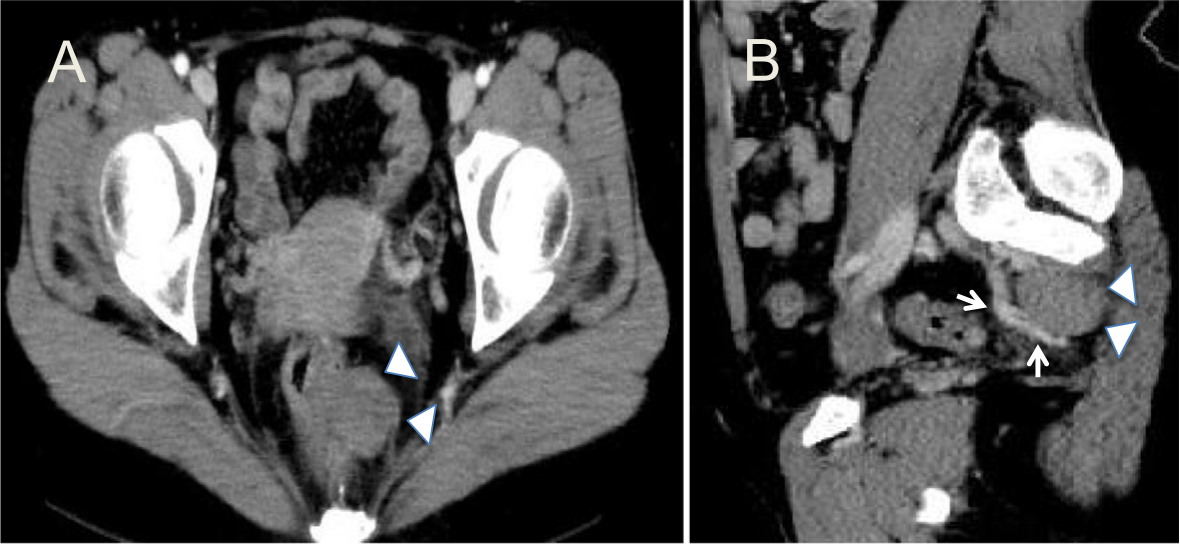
Contrast-enhanced computed tomography. a The multilocular cystic lesion has a diameter of approximately 32 mm, and no enhancing solid components or calcifications are visible (white arrowhead). b The blood supply arises from the middle sacral artery (white arrow)
